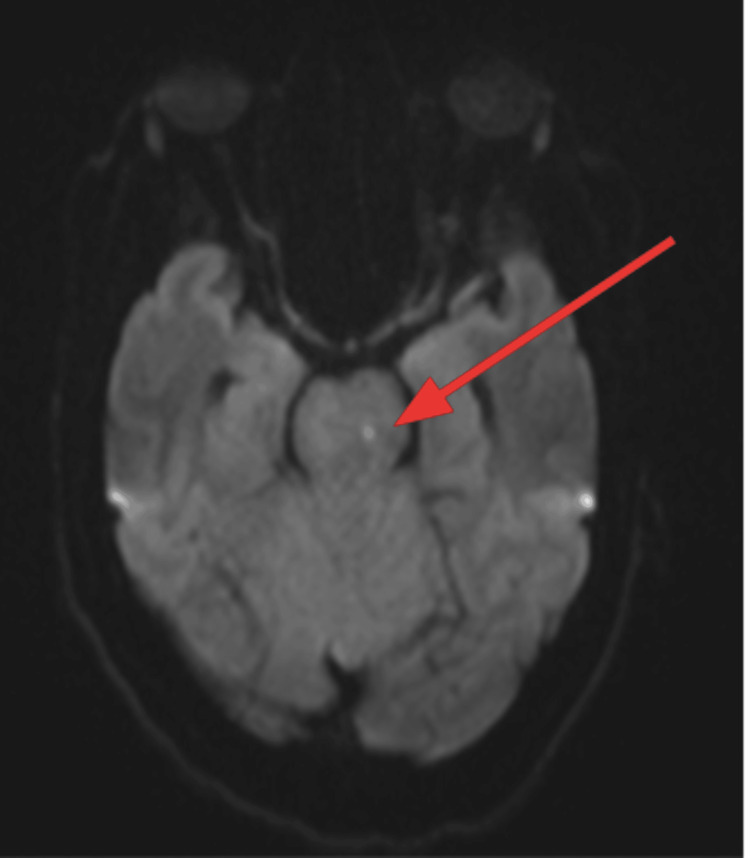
MRI of the brain T2 sequence (axial view) MRI of the brain T2 sequence showed a small hyperintense area in the left pons that correlated with the DWI sequence and ADC map, which was consistent with an acute pontine infarct (red arrow). MRI: Magnetic resonance imaging; DWI: Diffusion-weighted imaging; ADC: Apparent diffusion coefficient
